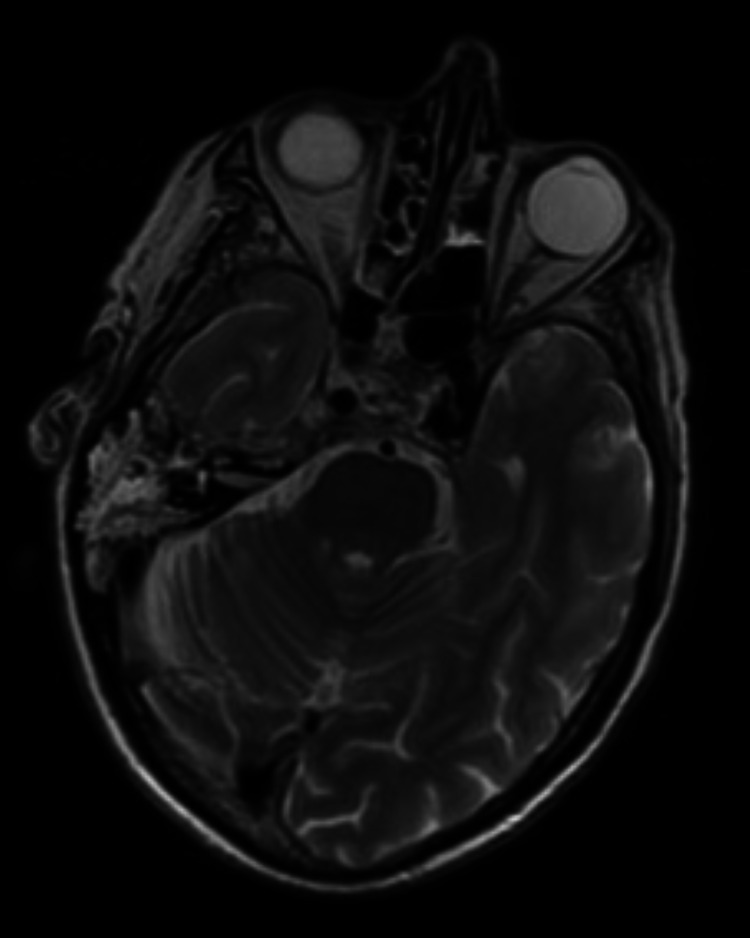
Initial MRI brain and neck of patient – T2-weighted.
